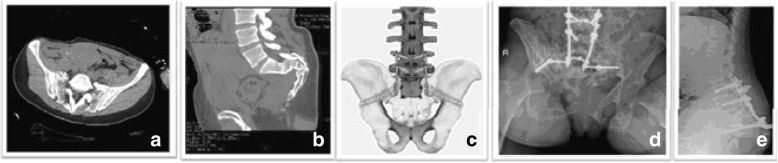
Case report: woman, 48 years. a-b H-shaped line fracture of the sacrum (Roy-Camille type III) and spinopelvic dissociation; (c) spinopelvic fixation [2]; d-e radiographic follow-up after 22 months
